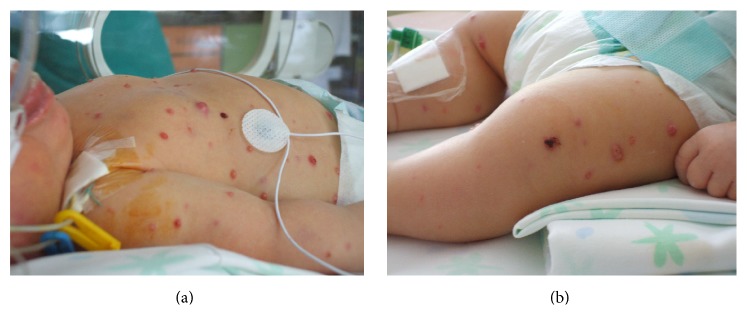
Nodular lesions diffused on the body in a term baby with alveolar rhabdomyosarcoma. Particular image of the chest and right arm (a) and the legs (b). An incisional biopsy of the cutis showed aggregates of atypical small spindle cells interrupted by fibrovascular septae and collagen bands resembling the lung alveoli. Bone marrow biopsy and total body CT scan demonstrated the presence of pulmonary, pleural, and pericardial metastasis. Chemotherapy was started, without a clinical improvement. The baby died at 26 days of life because of renal insufficiency and heart failure.
